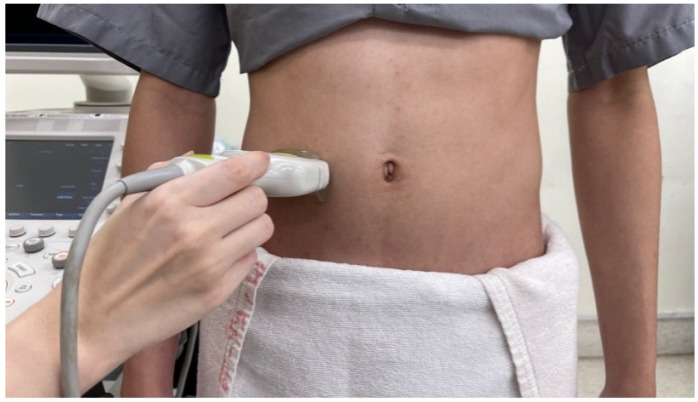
Location of TrA ultrasonographic.
